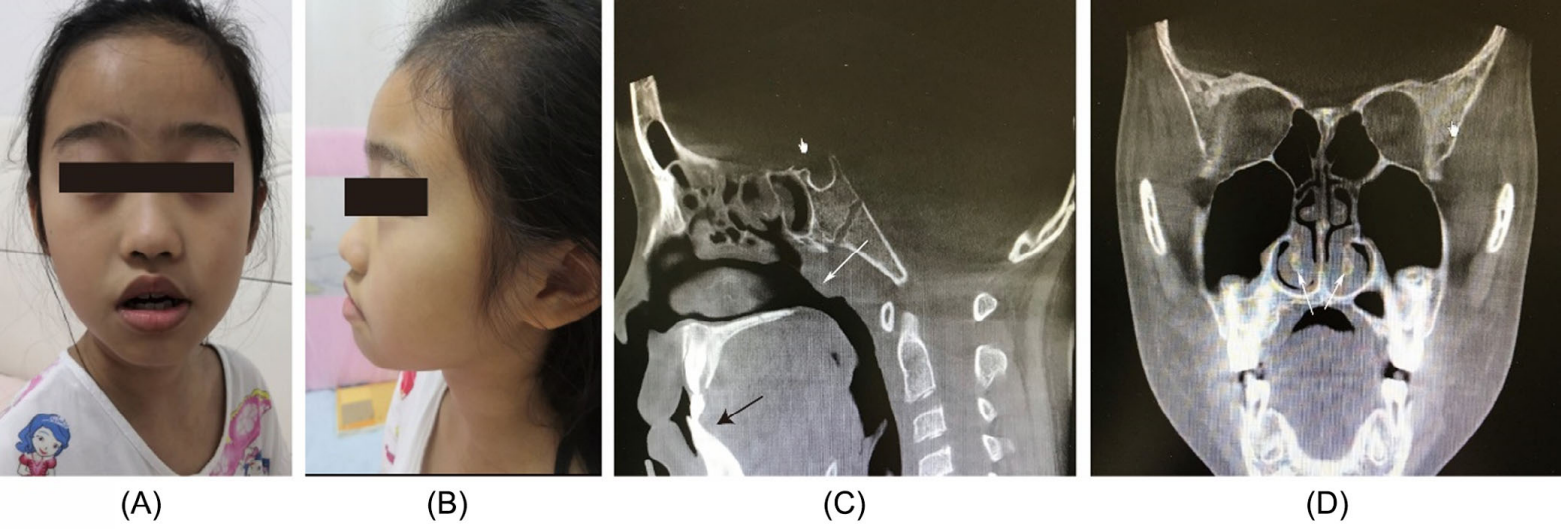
Data of the child in case 1. (A) “Adenoid face” of the child; (B) lateral view of the “adenoid face”. (C) CBCT sagittal reconstruction showing adenoid hypertrophy (white arrows) and dental retrusion (black arrows). (D) Coronal scan showing bilateral inferior turbinate hypertrophy (white arrows)
